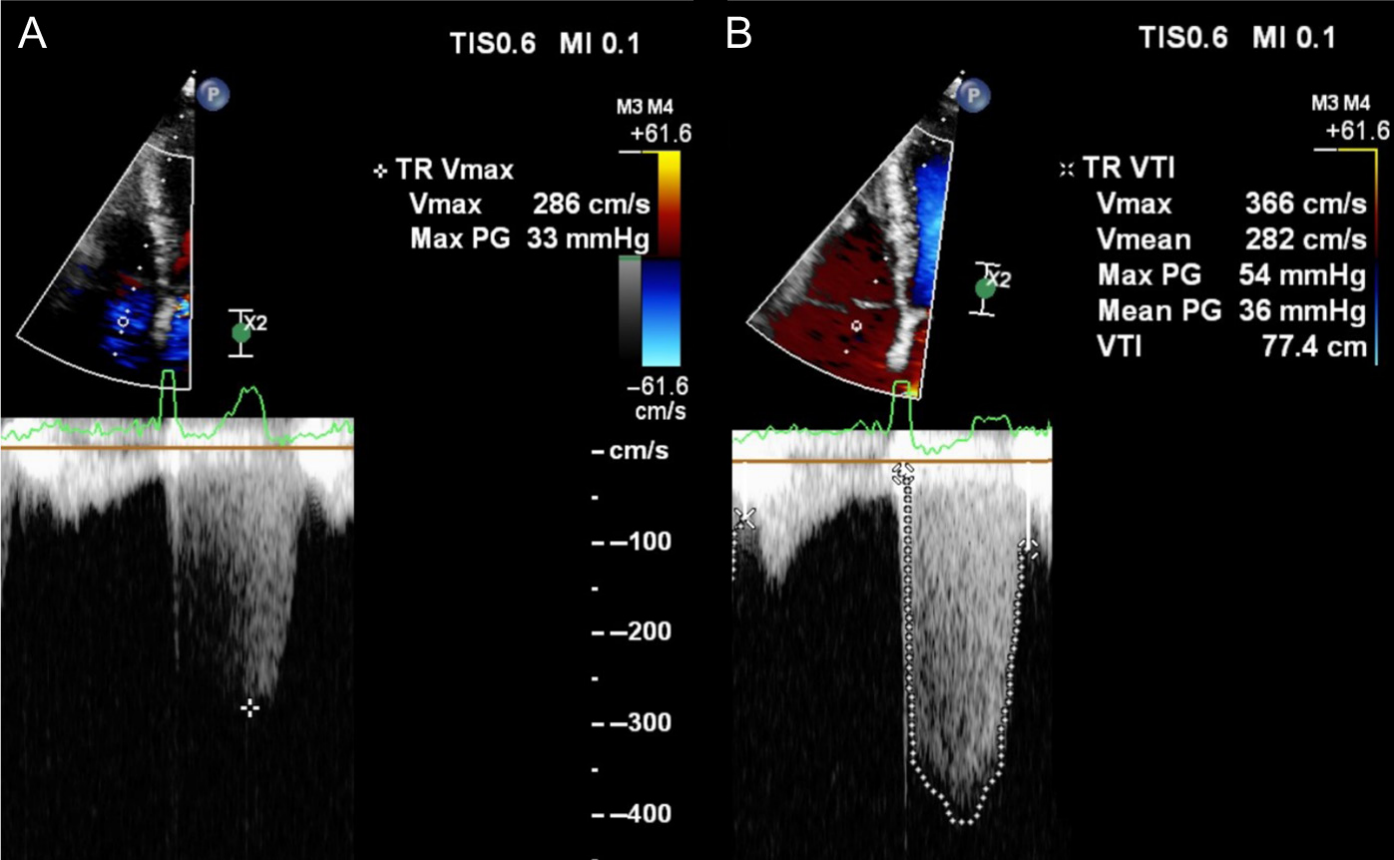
Continuous wave Doppler demonstrating an increase in severity of tricuspid regurgitation and increase in maximal velocity from rest (A) to exercise (B) while cycling at 75 W.
